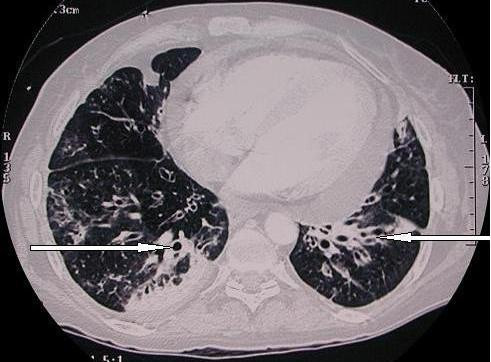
CT scan of the chest. Bronchial wall thickening and dilatation predominantly in the lower lung zones consistent with bronchiectasis can be seen.
